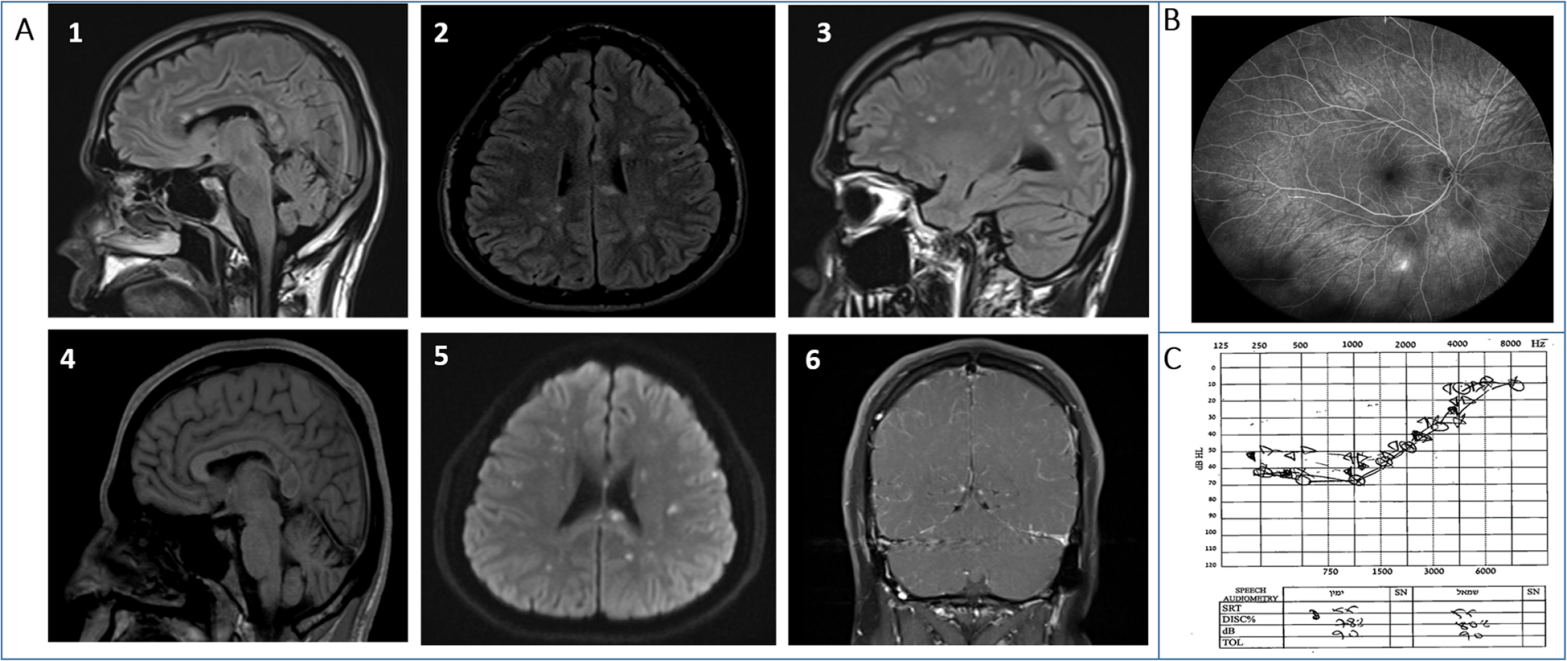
Typical diagnostic procedures findings. a Brain MRI. Sagittal and axial T2 FLAIR sequence, showing hyperintense lesions in the corpus callosum (“snow balls”). A_1_. Periventricular and subcortical areas. A_2-3_. Sagittal T1 sequence corpus callosum (“black holes”). A_4_. Axial DWI sequence which show restricted diffusion A5. Leptomeningeal enhancement with gadolinium A_6_. b Wide field Fluorescein angiogram demonstrating right eye peripheral (superior, temporal and inferior) branch artery occlusions and focal arterial leakage in an inferior branch artery. c. A bilateral low-tone and middle-tone sensorineural hearing loss is seen, with abnormal low scores in speech audiometry
